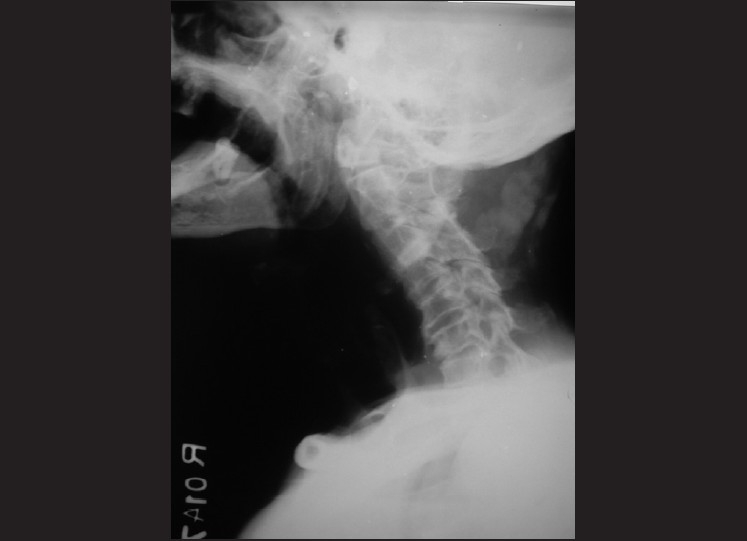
X-ray of the nasopharynx lateral view revealed the radiopaque foreign body in the naso-oropharynx
